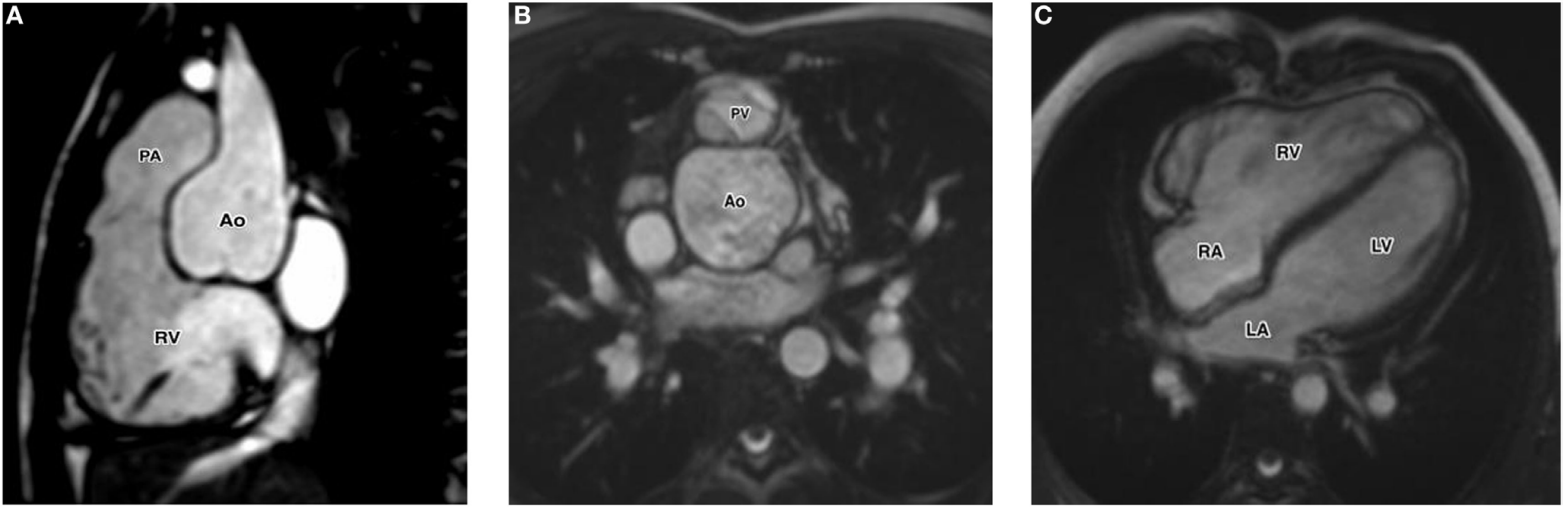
(A) Cardiac MRI with sagittal view of the right ventricular outflow tract showing the anterior leaflet of the pulmonary valve without attachment to the annular hinge. (B) Cardiac MRI with axial view of the pulmonary valve showing morphologic abnormality of the anterior leaflet. (C) Cardiac MRI with four-chamber views showing the dilatation of the right ventricle. Abbreviation: LA, left atrium; LV, left ventricle; RA, right atrium; RV, right ventricle.
